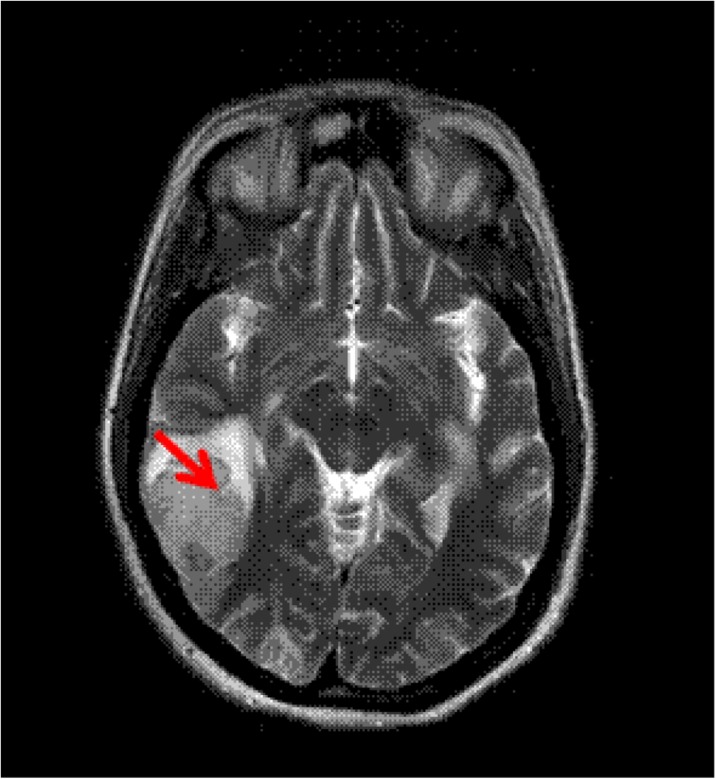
MRI- PRES with intraparenchymal hemorrhage
